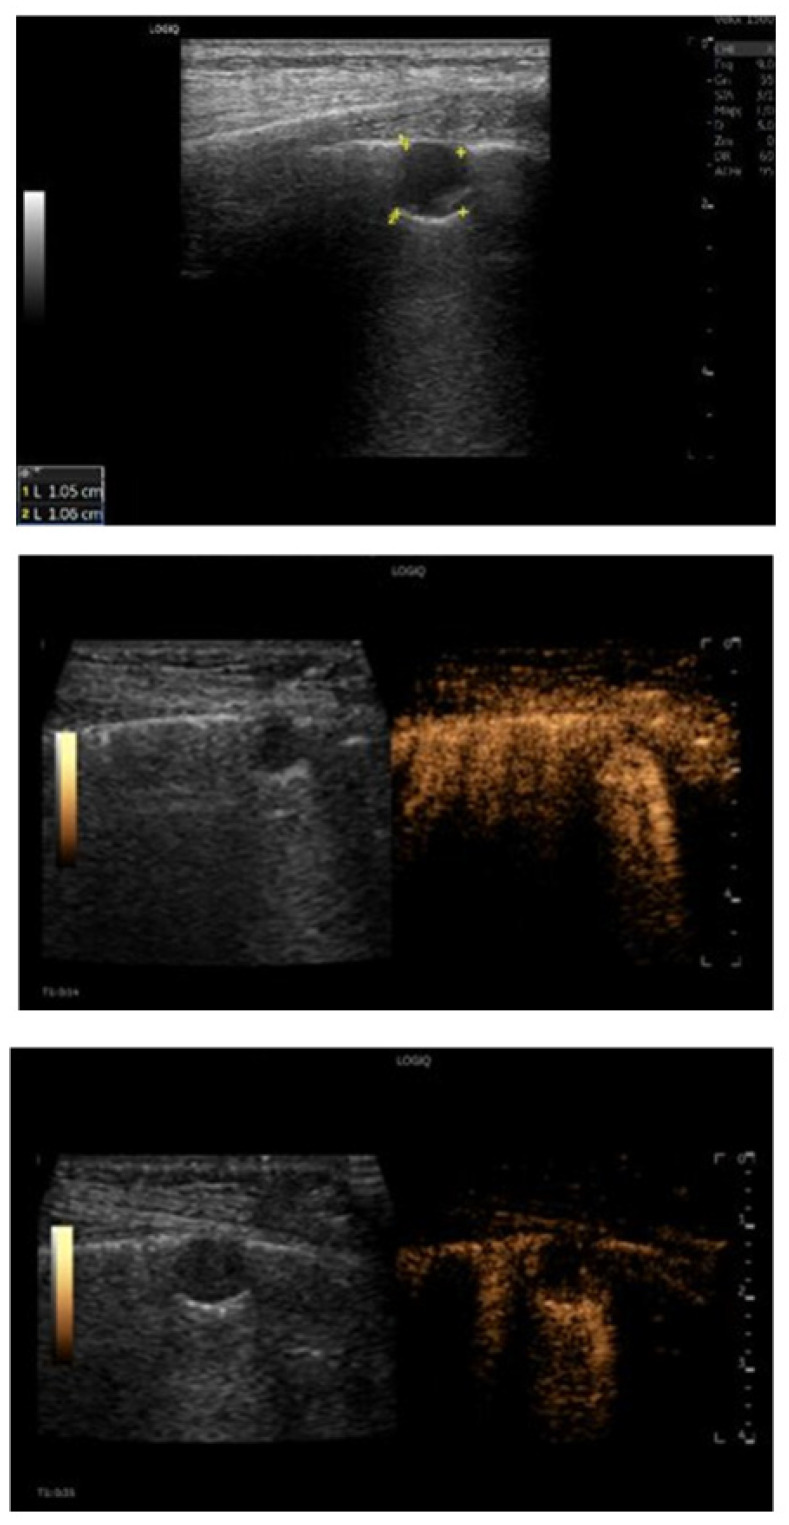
B-mode and contrast-enhanced ultrasound (CEUS) features of a peripheral pulmonary metastasis from breast carcinoma. Ultrasound demonstrates a small, oval-shaped peripheral pulmonary lesion showing early contrast enhancement followed by rapid and complete wash-out, a perfusion pattern consistent with metastatic disease. Final diagnosis was pulmonary metastasis from breast carcinoma.
